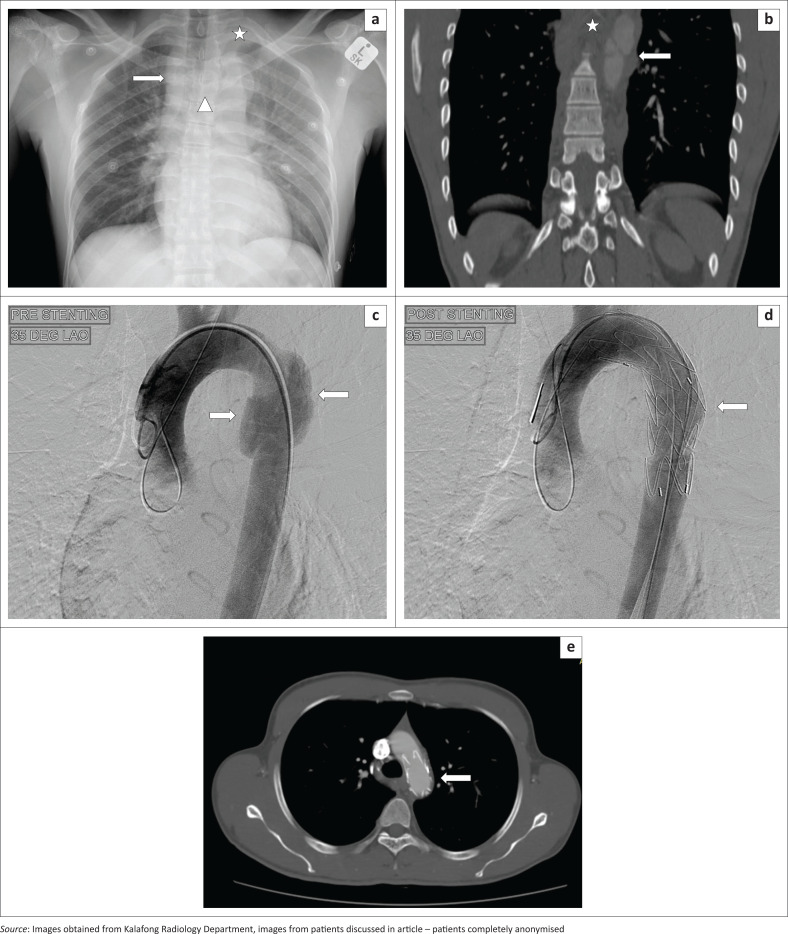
(a) Chest radiography in a 36-year-old male patient involved in a motor vehicle accident. There is widening of the mediastinum (arrow) with a left apical pleural cap (star) and veiling of the left lung field in keeping with a left haemothorax. There is depression of the left main bronchus (triangle), and the silhouette of the aortic knuckle is maintained. (b) Computed tomography angiography demonstrating a mediastinal haematoma (star) and a type 2 aortic injury with an intimal flap and a pseudoaneurysm (arrow). (c) Digital subtraction angiogram image prior to stenting delineating the false aneurysm (arrows) beyond the origin of the left subclavian artery. (d) Digital subtraction angiogram image post successful endovascular aneurysm repair with the stent in place (arrow). (e) Follow-up computed tomography angiography indicates the stent in place with no complications (arrow).
